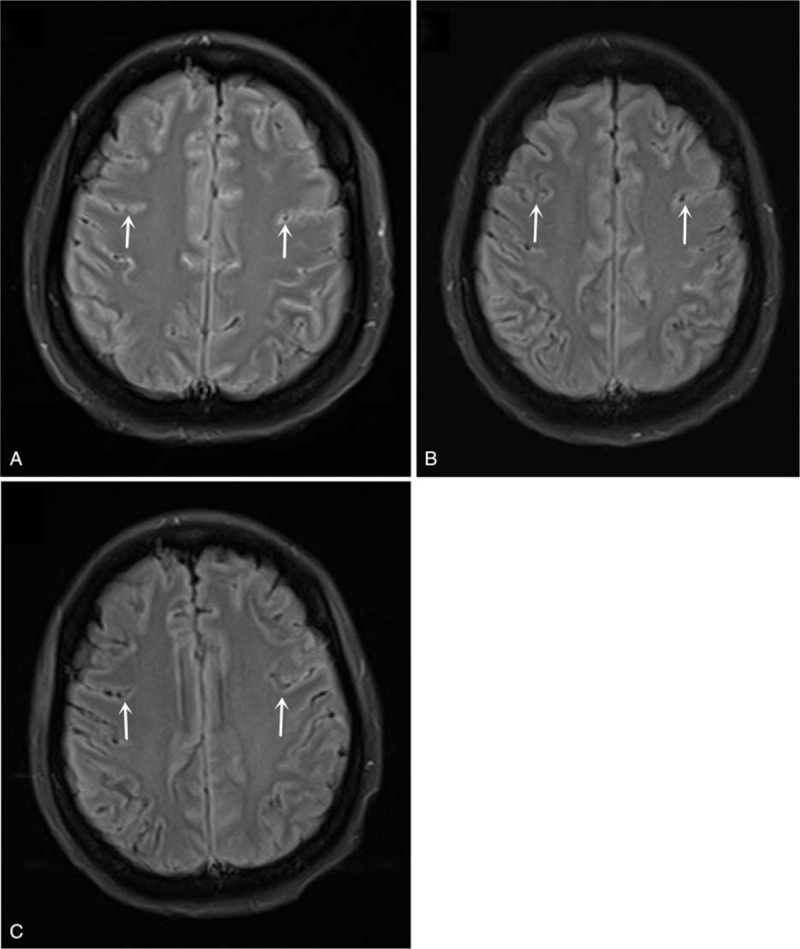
Cranial MRIs. A, Enhanced MRI scan performed on April 01, 2019 with slightly abnormal signal enhancement in the pons, indicative of brain metastasis. Linear FLAIR signals were present around the brainstem, bilateral cerebral hemispheres, and cerebellar sulci, which was indicative of soft meningeal metastasis. B, Enhanced MRI scan performed on May 15, 2019 with linear FLAIR signals around the brainstem, bilateral cerebral hemispheres, and cerebellar hemispheres in the sulci, suggesting soft meningeal metastasis. The range was smaller than that seen on the previous MRI. C, Enhanced MRI scan performed on June 19, 2019 indicating few linear FLAIR signals in the left parietal and right parietal localized sulci. The range was smaller than that seen on the previous MRI.
